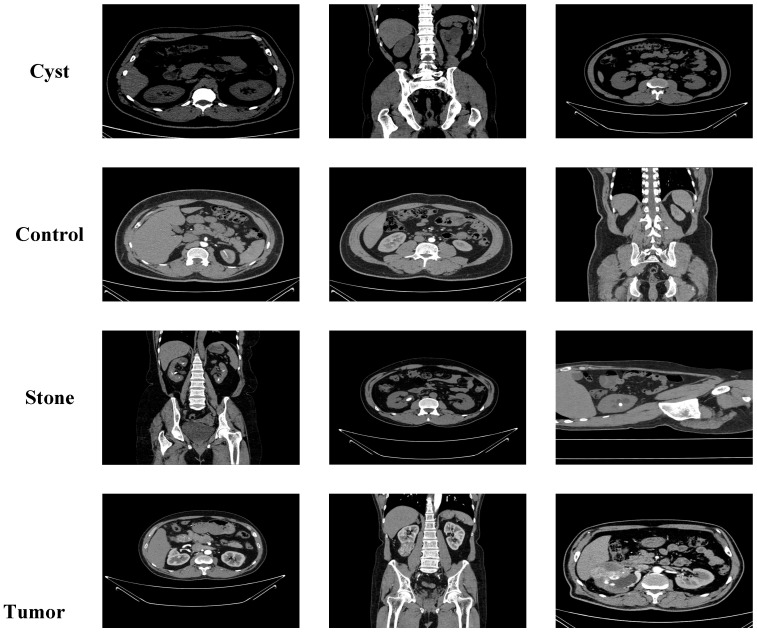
Sample images from the Kaggle CT KIDNEY Dataset.
